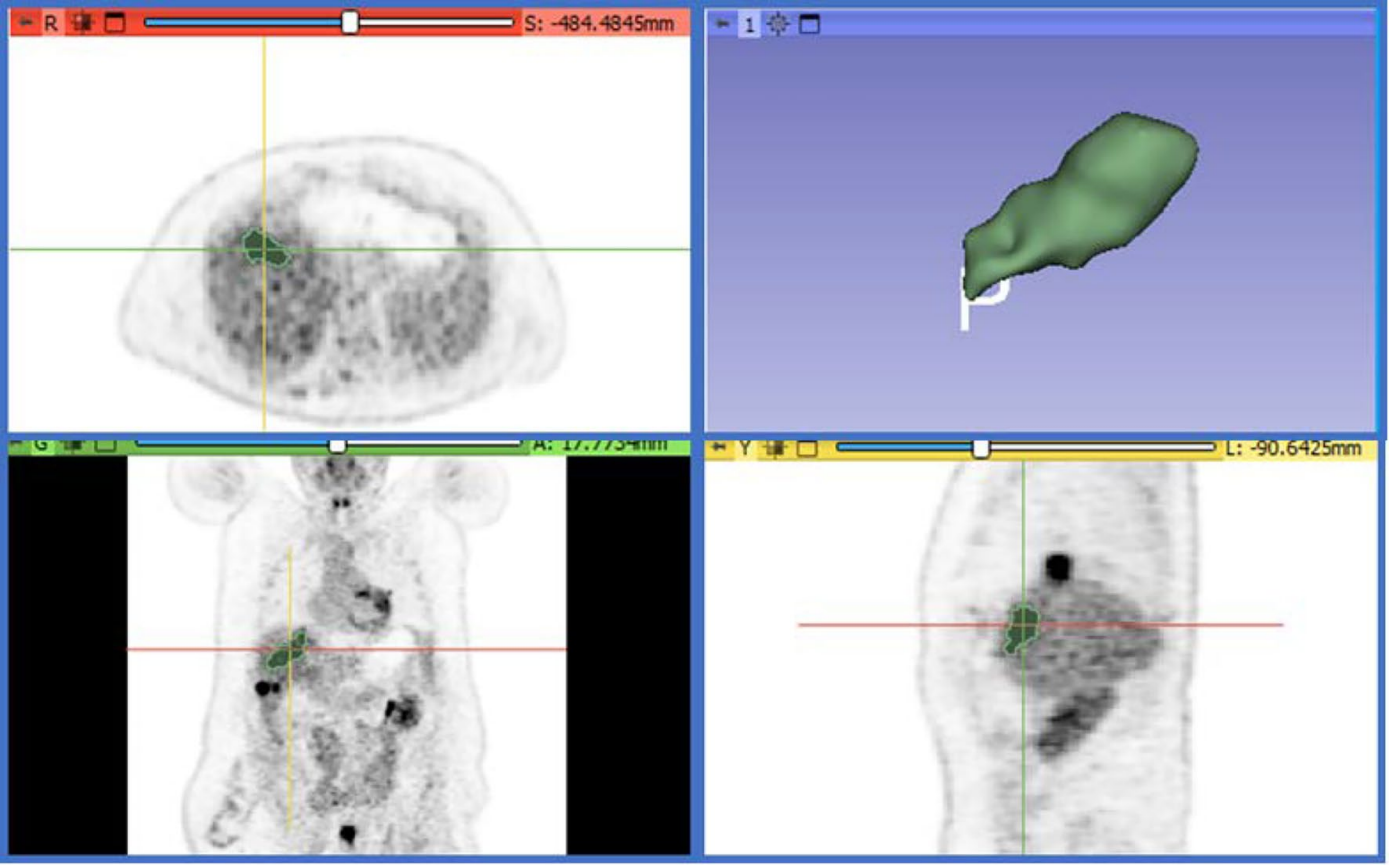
Tumor segmentations. All tumor segmentations were performed using 3D Slicer software. All ^18^F-FDG PET images were converted to SUVbw adjusted values. The largest lesions in the procedure lobe were first segmented automatically using the threshold method. Tumors with SUVbw greater than 4 were automatically selected within the largest tumor. In instances when there are adjacent tumors and automatic segmentation yielded unsatisfactory results, such as overlapping borders with adjacent tumors, manual corrections were made through three different planes. Finally, the segmented volume underwent radiomic feature extraction. *SUVbw* Standardized Uptake Values body-weight adjusted
